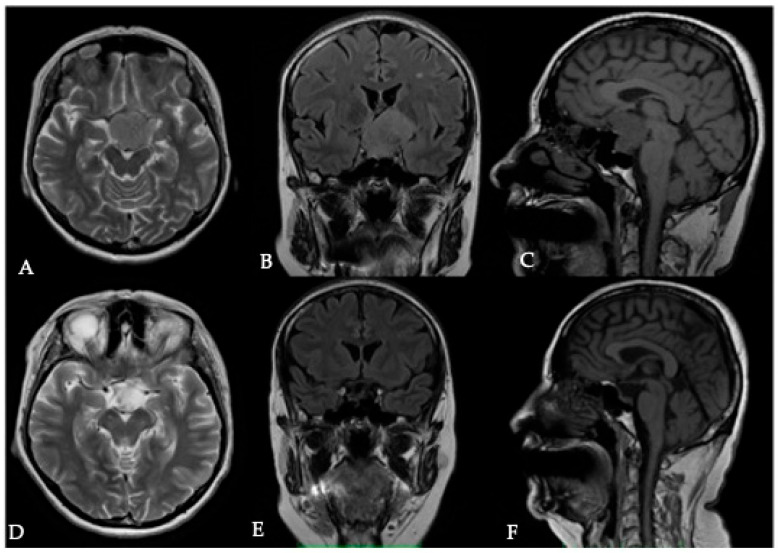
Pre- and postoperative MRI images of a patient with a tuberculum sellae meningioma (TSM). The preoperative images demonstrate hyperintensity on T2-weighted imaging (A) and T2 FLAIR (B) along with hypointensity in T1WI (C), indicative of a soft tumor, which facilitates complete removal. Postoperative MRI sections (D–F), shown below, confirm complete tumor resection.
